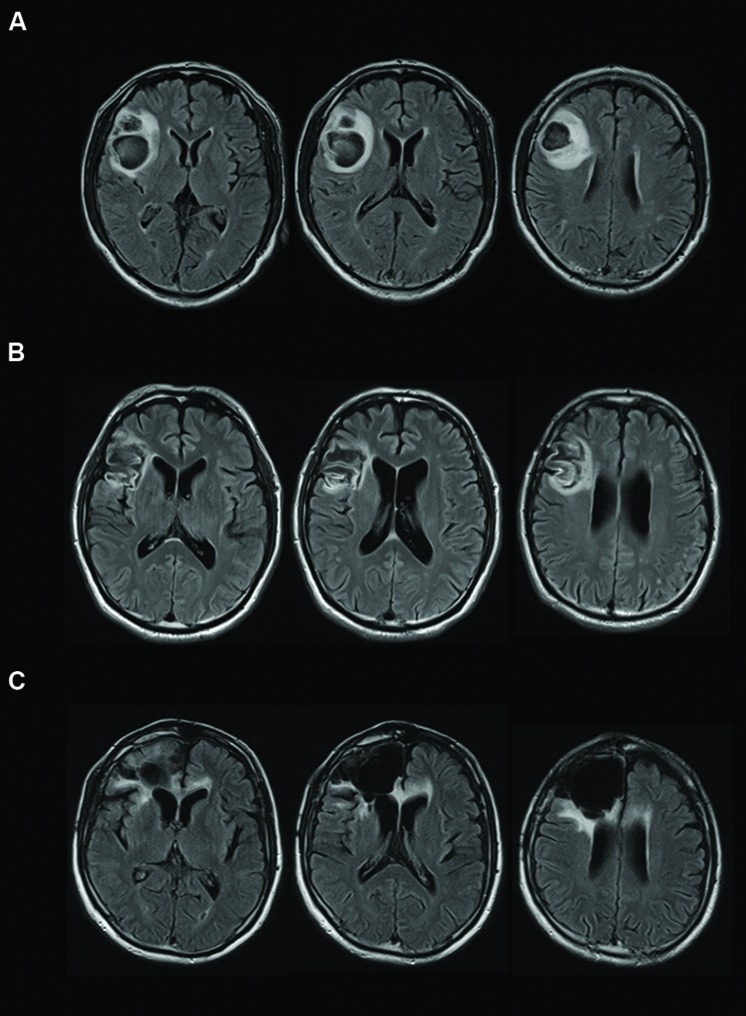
Transverse magnetic resonance imaging with FLAIR acquisition of the brains of the cases. Left sides of images correspond to the right side of the brain. (A) Case A, (B) Case B, and (C) Case C.
